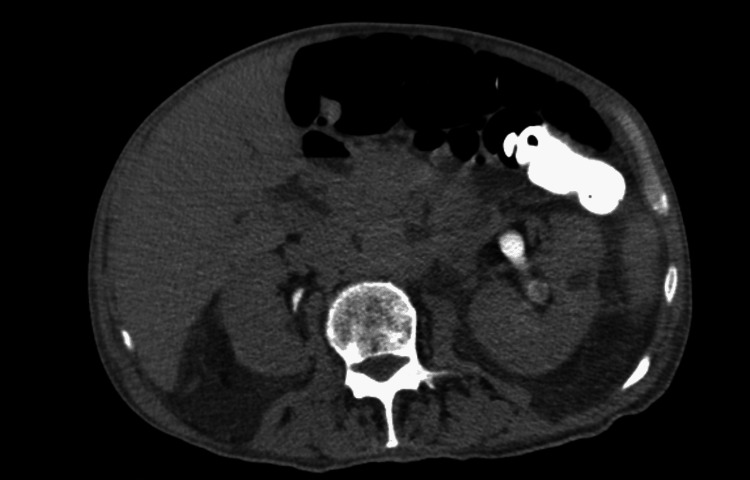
Kidneys Abdominal computed tomography (CT) scan without intravenous contrast medium. Normal kidneys.
